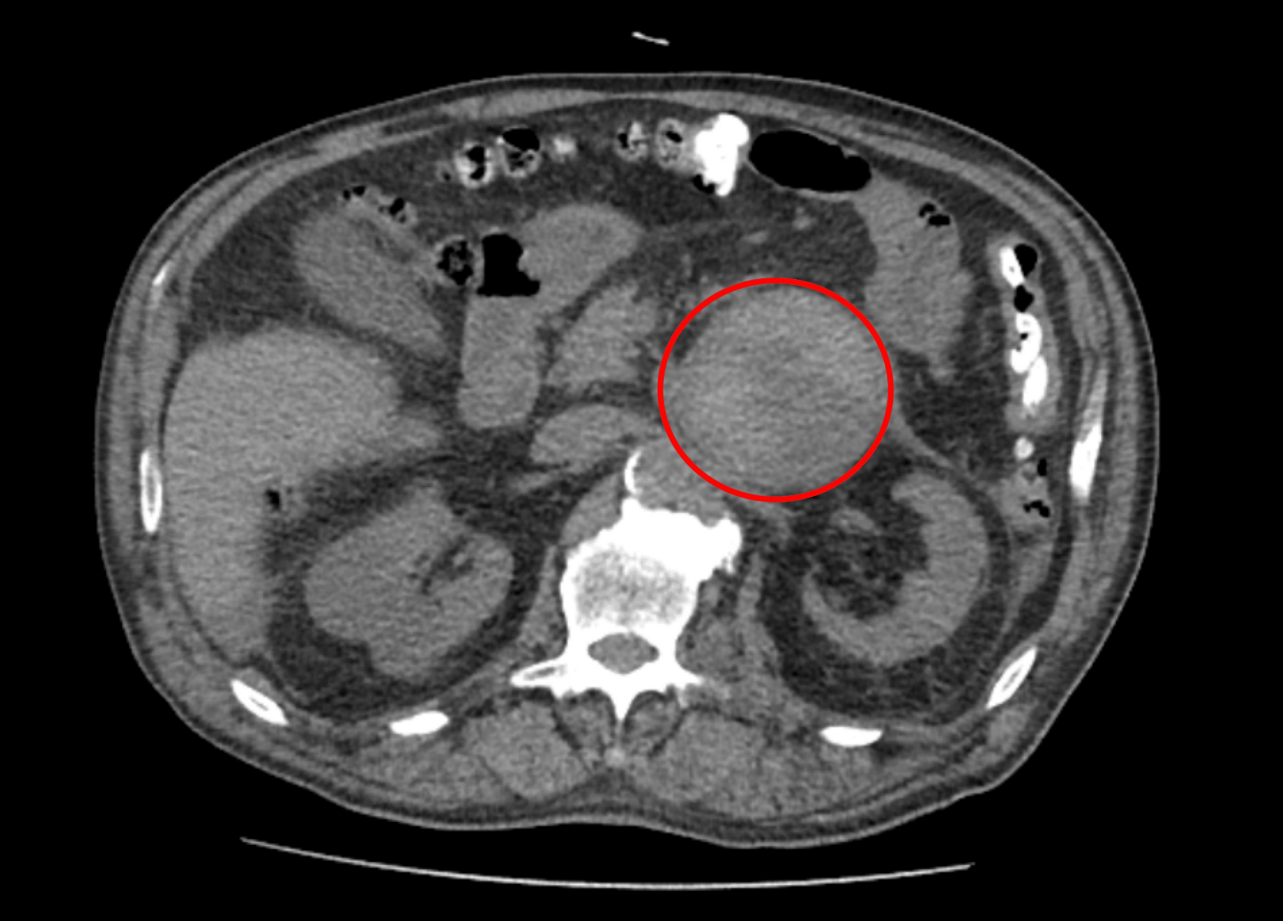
Computed tomography at discharge (without contrast): lesion with slight reduction in size and more homogeneous density, suggestive of haemorrhage in the resolution phase
